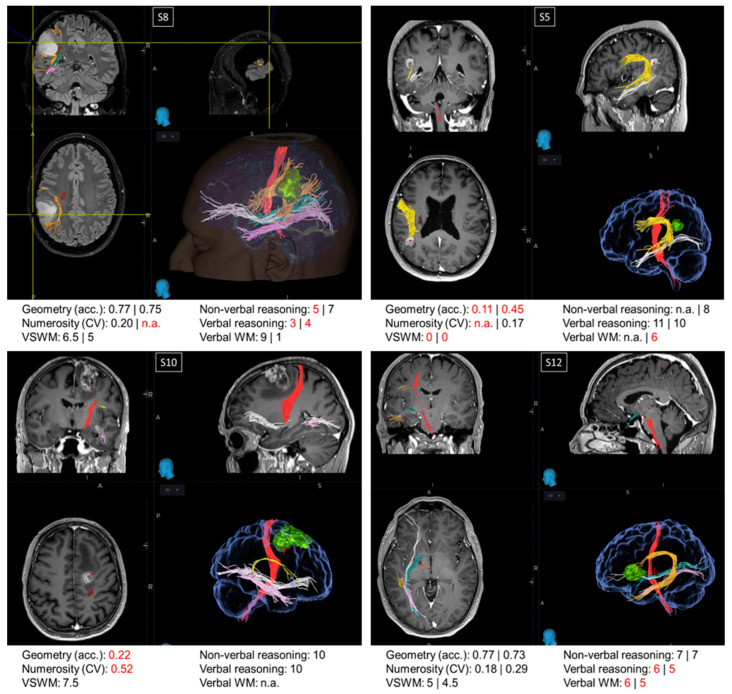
Single cases. Images from the NeuroNavigation system, Medtronic (reading left image_left side/right image_right side): MRI images displaying the lesions and scores in neuropsychological and psychophysical tests in patients S8, S5, S10, and S12. When two values are present, the first refers to the score before the surgery and the second refers to the score obtained after the surgery. Scores below the normative value are marked in red. Major fibers obtained by MRI DTI before the surgery are color-coded as follows: red: corticospinal tract; orange/yellow: arcuate fasciculus; green: optic radiations; pink: inferior longitudinal fasciculus (ILF); white: inferior fronto-occipital fasciculus (IFOF).
